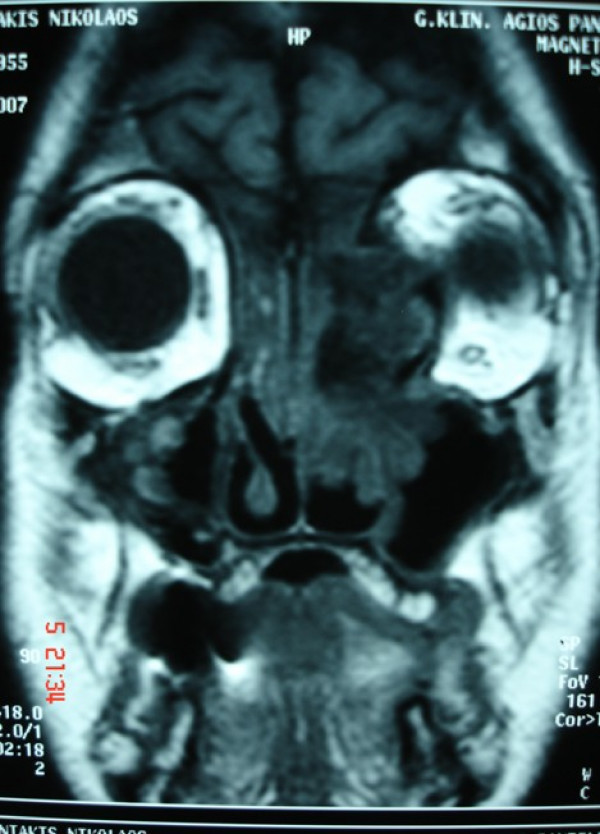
Preoperative coronal T1-weighted magnetic resonance image reveals a mass with lateral displacement of the left orbital contents and attachment of the tumor to the anterior skull base without intracranial involvement.
